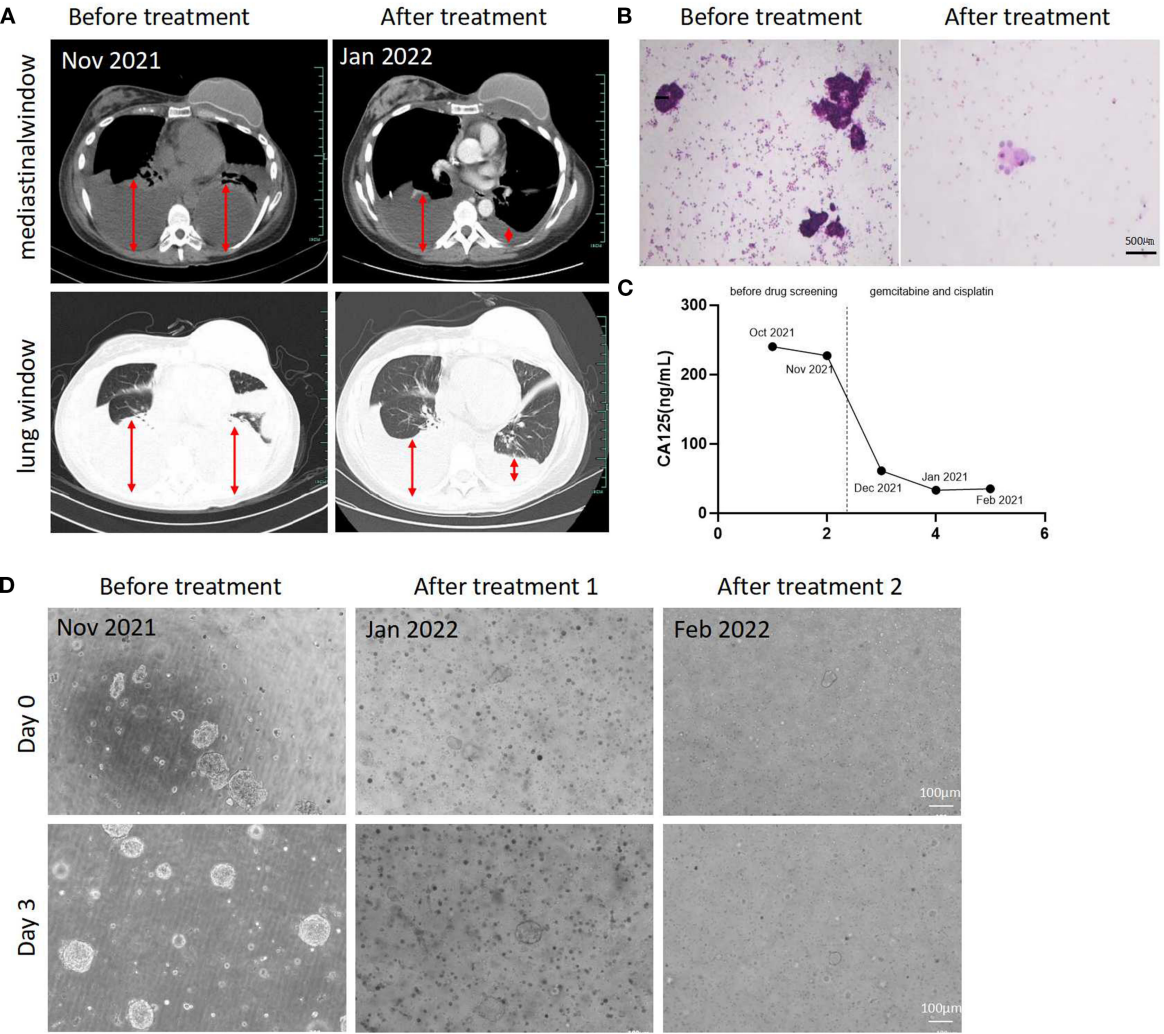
Evaluation of the effectiveness of drug guided by Organoid drug sensitivity tests. (A) CT images of this patient before and after Gemcitabine and Cisplatin treatment. (B) Cytological pathological images of thoracic pleural effusion before and after the initiation of Gemcitabine and Cisplatin therapy. Scale bar = 500 μm. (C) Curve of CA125 level changes in peripheral blood. (D) Images of organoid growth before and after Gemcitabine and Cisplatin treatment. Scale bar = 100 μm.
